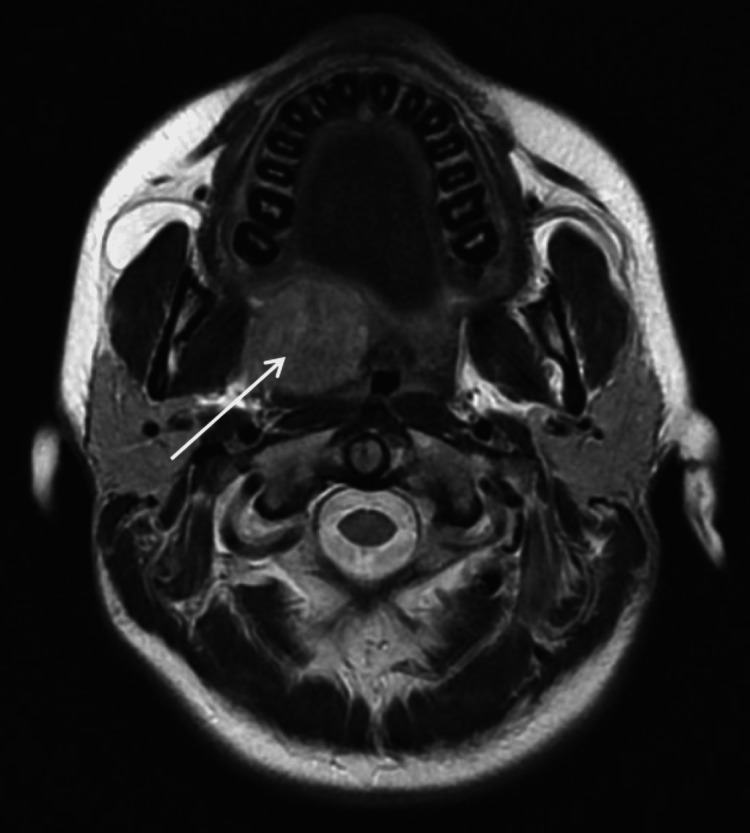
Magnetic resonance imaging showing a heterogeneous mass on the right side of the soft palate in T2 (arrow), well limited without extension to the surrounding tissues
